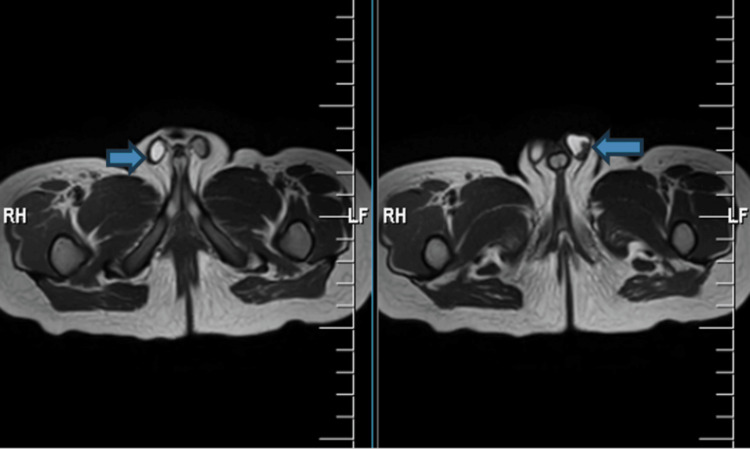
Presence of bilateral testes on the MR T2WI axial section of the pelvis MR T2WI: magnetic resonance T2-weighted image
